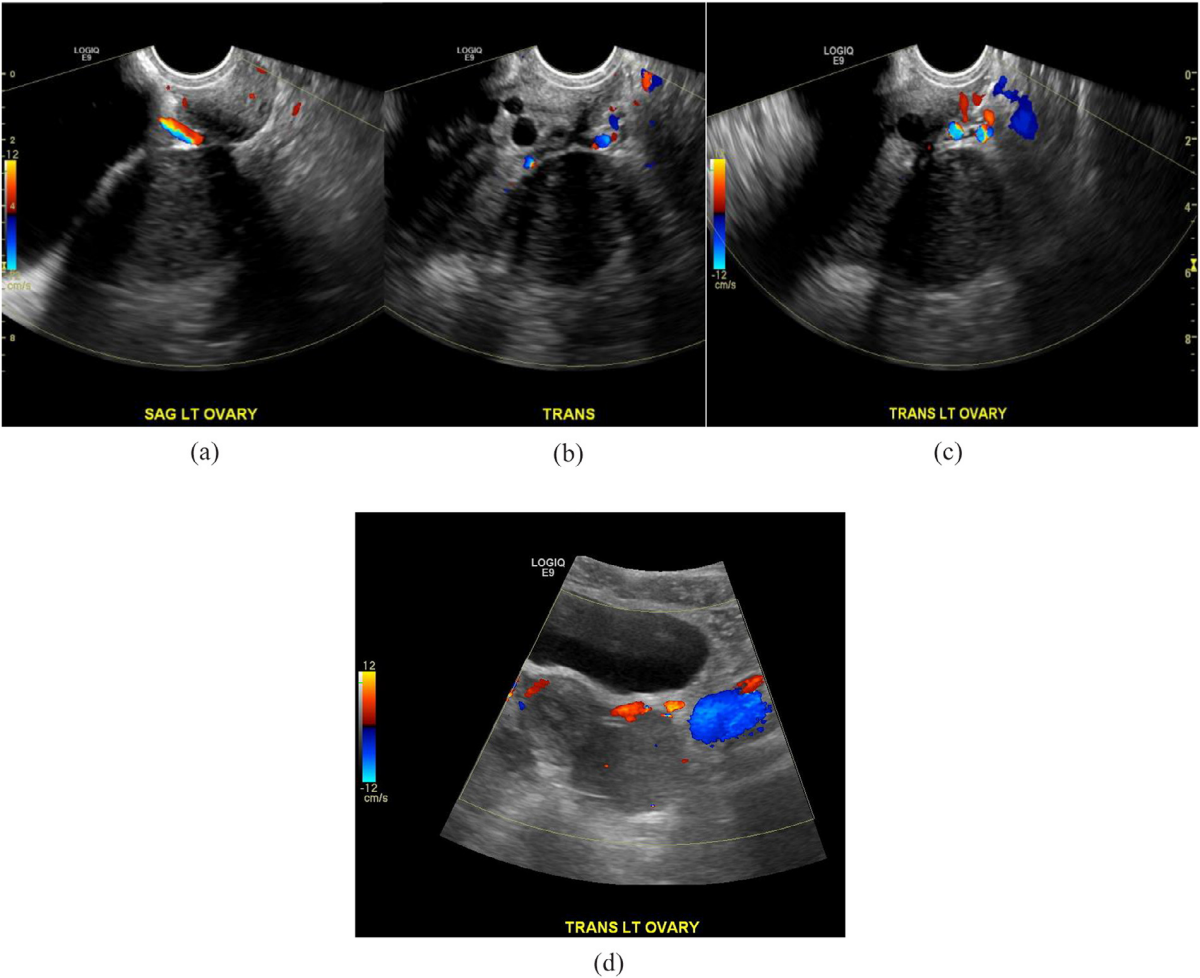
(A-C) Transvaginal ultrasound of left ovaria showing no significant flow. (D) Transabdominal ultrasound of the left ovary showing very minimal flow.
